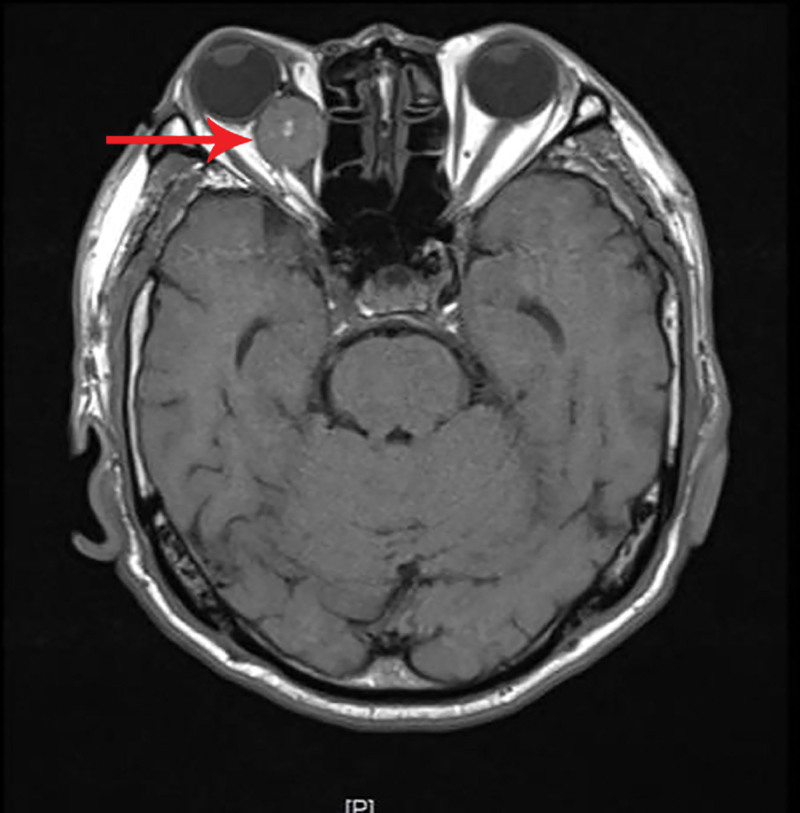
T1-weighted magnetic resonance imaging showing a retrobulbar mass with focal intralesional hyperintensity (arrow).
